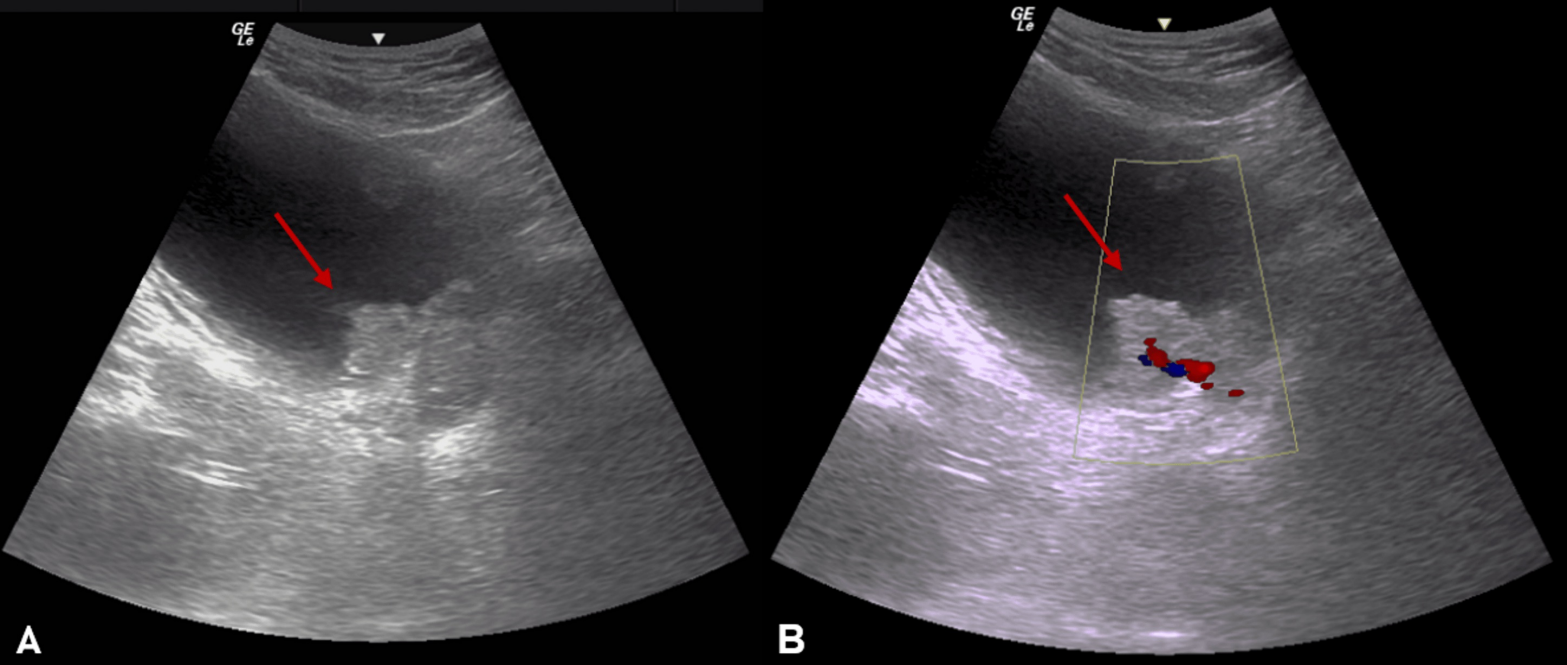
Ultrasonography identifying a solitary papillary lesion (A) Gray-scale sagittal ultrasound demonstrates a well-defined hyperechoic intravesical mass arising from the left posterior wall (red arrow); (B) Color Doppler confirms internal vascular flow within the lesion (red arrow).
